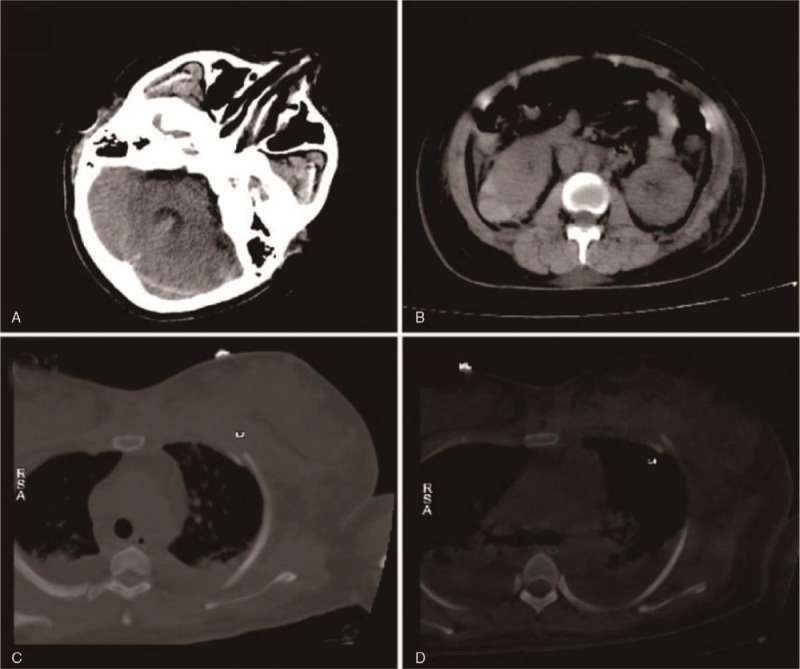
Computed tomography showing subarachnoid hemorrhage and right renal capsule hematoma.
